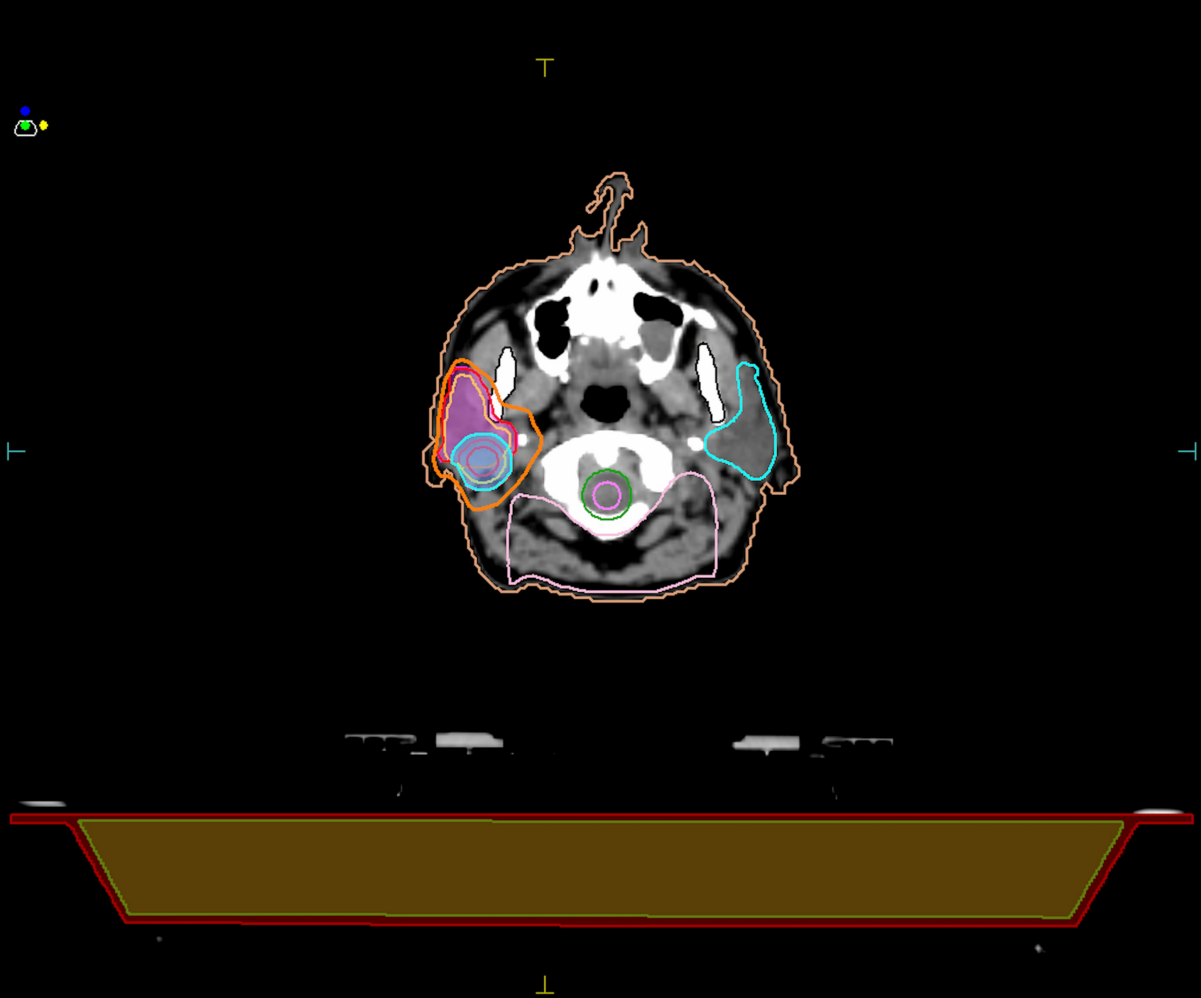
CT-based RT treatment planning showing a transverse (axial) slice at the level of the parotid glands. The RT regimen employed a SIB approach, delivering a total dose of 70 Gy to the right parotid tumor bed in 35 fractions, with a daily dose of 2 Gy per fraction. Concurrently, regions identified with high-risk lymph nodes received 63 Gy, also administered in 35 fractions at 1.8 Gy per fraction, while elective lymph node areas were treated with 56 Gy, delivered in 35 fractions with a daily dose of approximately 1.6 Gy per fraction. CT: computed tomography; RT: radiation therapy; SIB: simultaneous integrated boost
